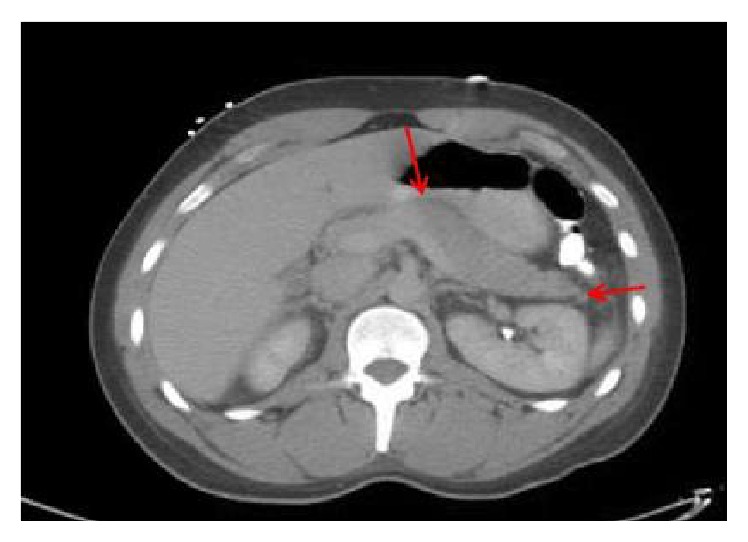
CT of the abdomen with contrast showing swollen pancreas and peripancreatic stranding.
